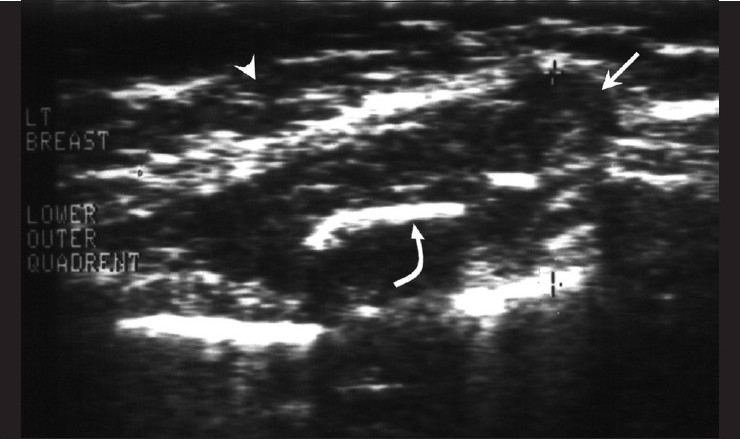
Rib tuberculosis – patient 10. Ultrasonography of the lateral quadrant of the left breast (arrow head) shows a hypoechoic collection (arrow) within which echogenic bone fragments of destroyed ribs (curved arrow) are seen.
